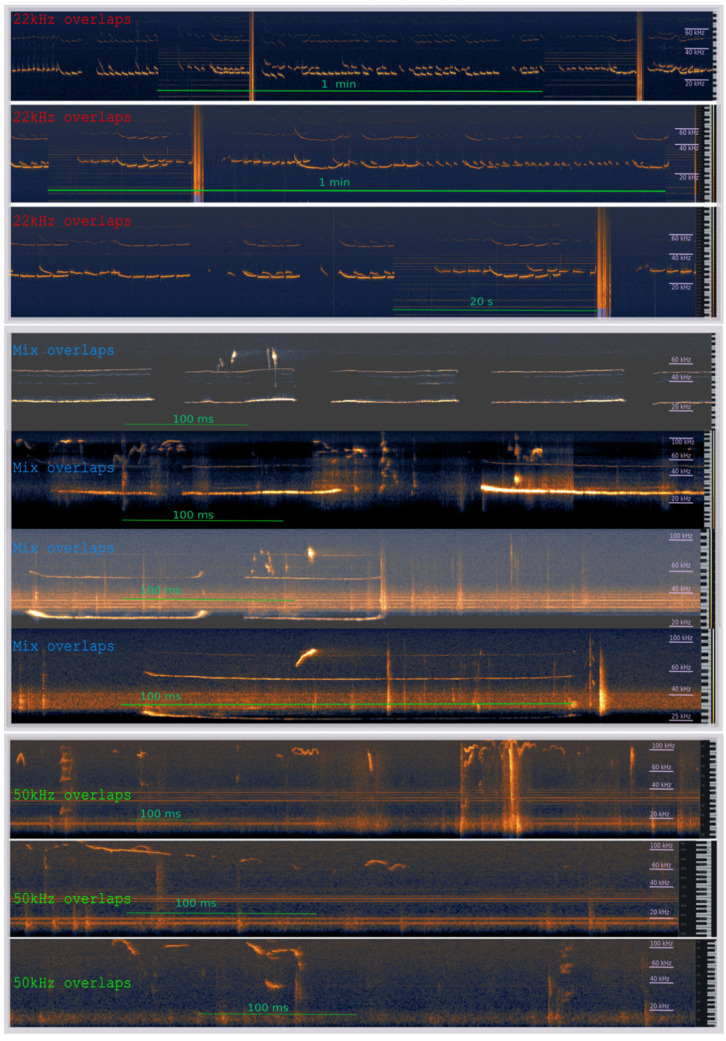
An example of overlapping ultrasonic vocalization presented on a spectrogram.
